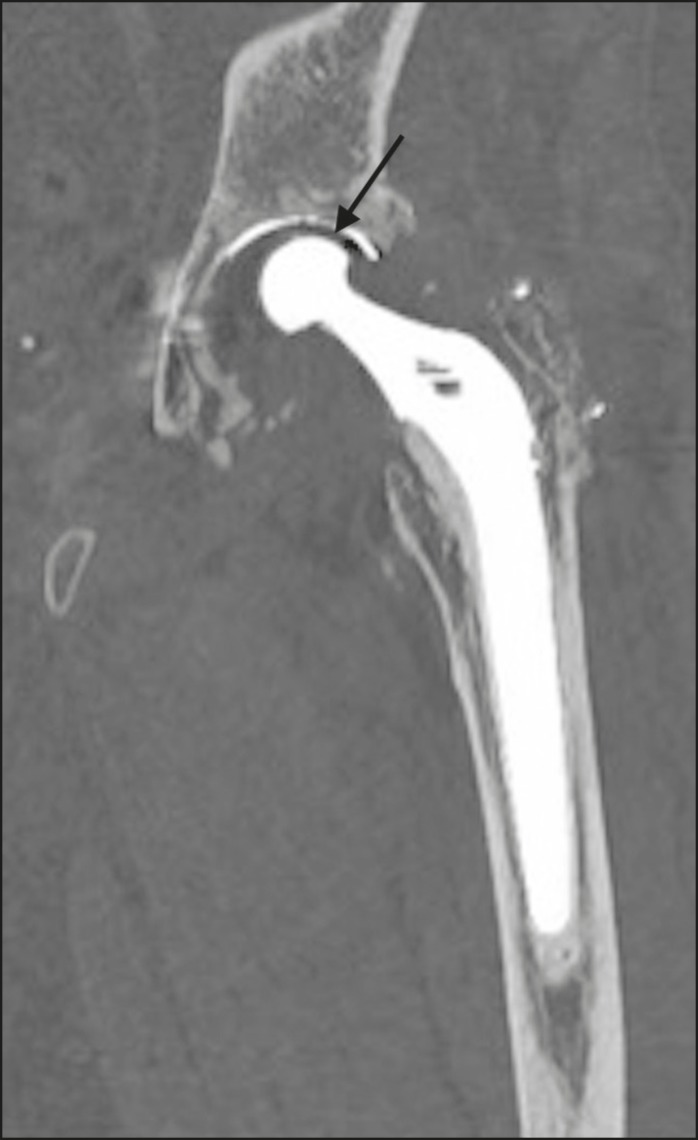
Computed tomography of the left hip in the coronal plane. Total left hip arthroplasty with reduction of the upper space, between the metallic head and acetabular components (arrow), indicating wear of the polyethylene lining.
